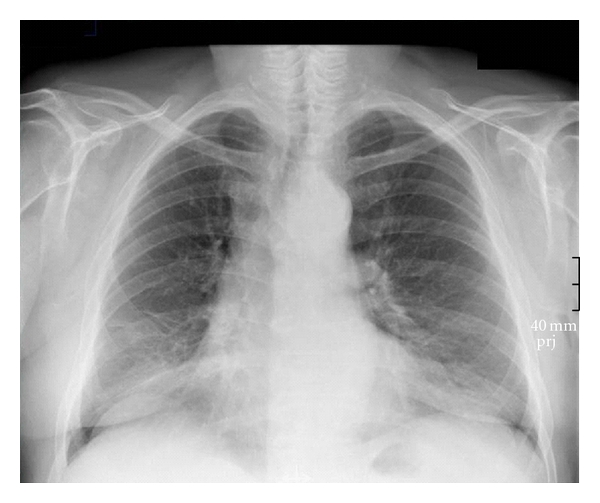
Chest radiograph seven months after bronchoscopic resection.
